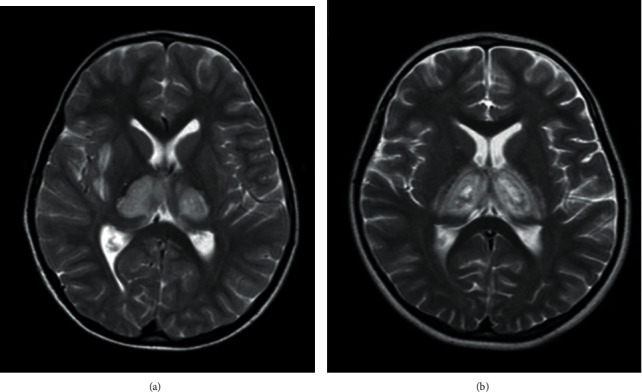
MRI images of case 2 (a) and case 3 (b).
